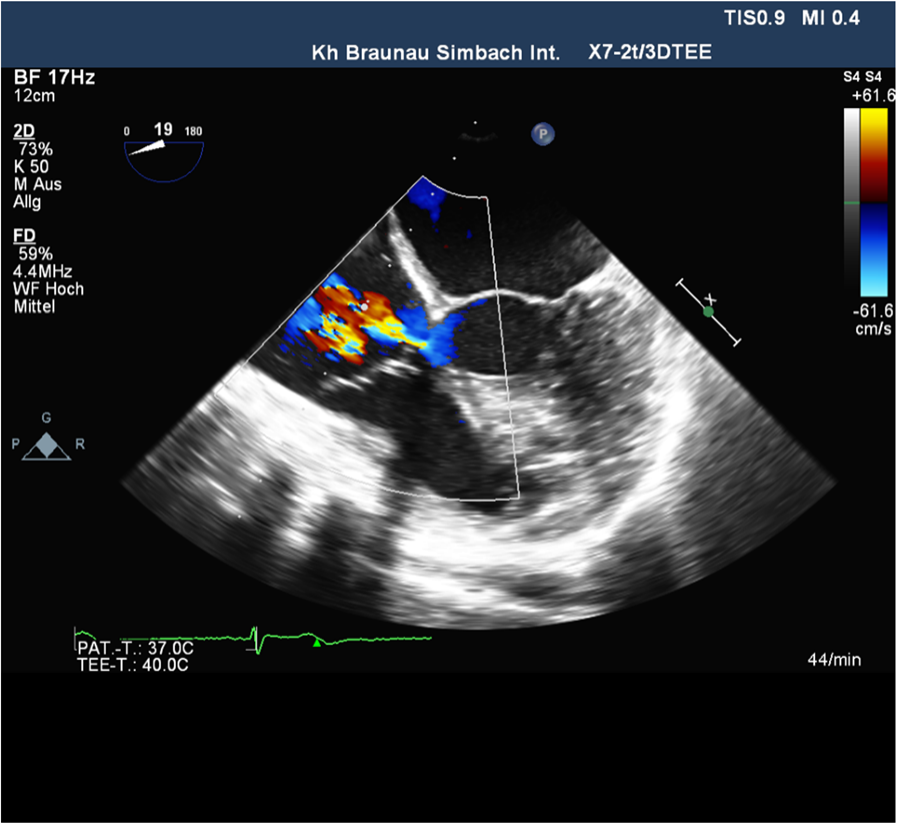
Two-dimensional transesophageal echocardiogram shows the Gerbode defect (arrows) in apical 4-chamber view.
